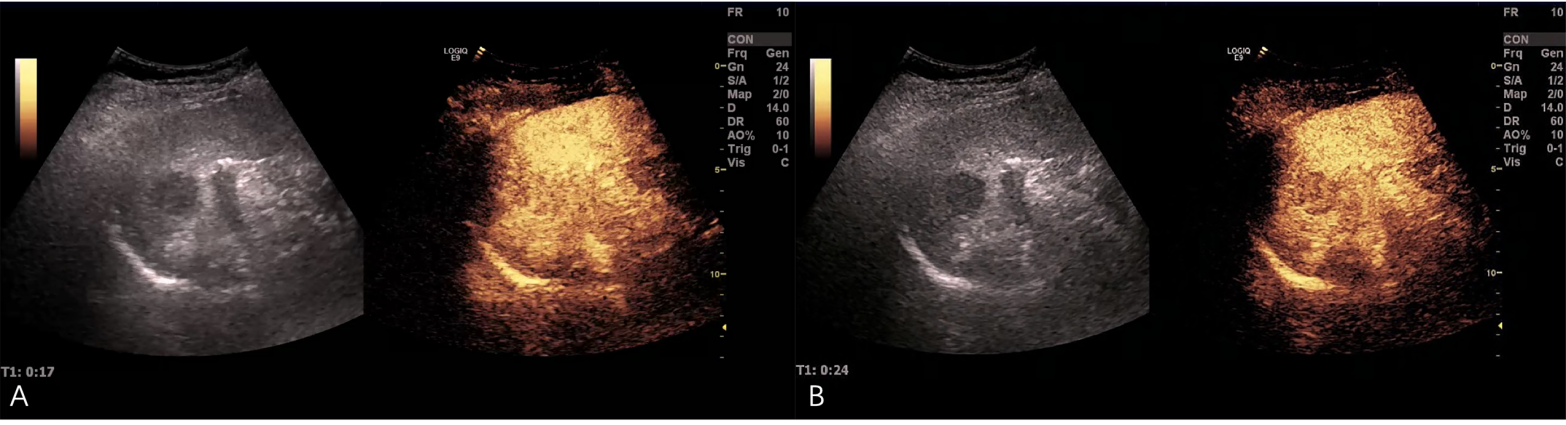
Contrast-enhanced ultrasound (CEUS) images. (A) After the infusion of the contrast agent SonoVue, the mass at the splenic hilum began to show inhomogeneous enhancement (low enhancement) in the arterial stage, and the enhanced edge was clear. The enhancement reached the peak at 17 s, and the peripheral splenic tissue showed uniform enhancement as compared to the mass. (B) The mass began to show a decline in enhancement at 24 s in the venous stage. The tumor mass showed delayed regression as compared to the surrounding tissue.
